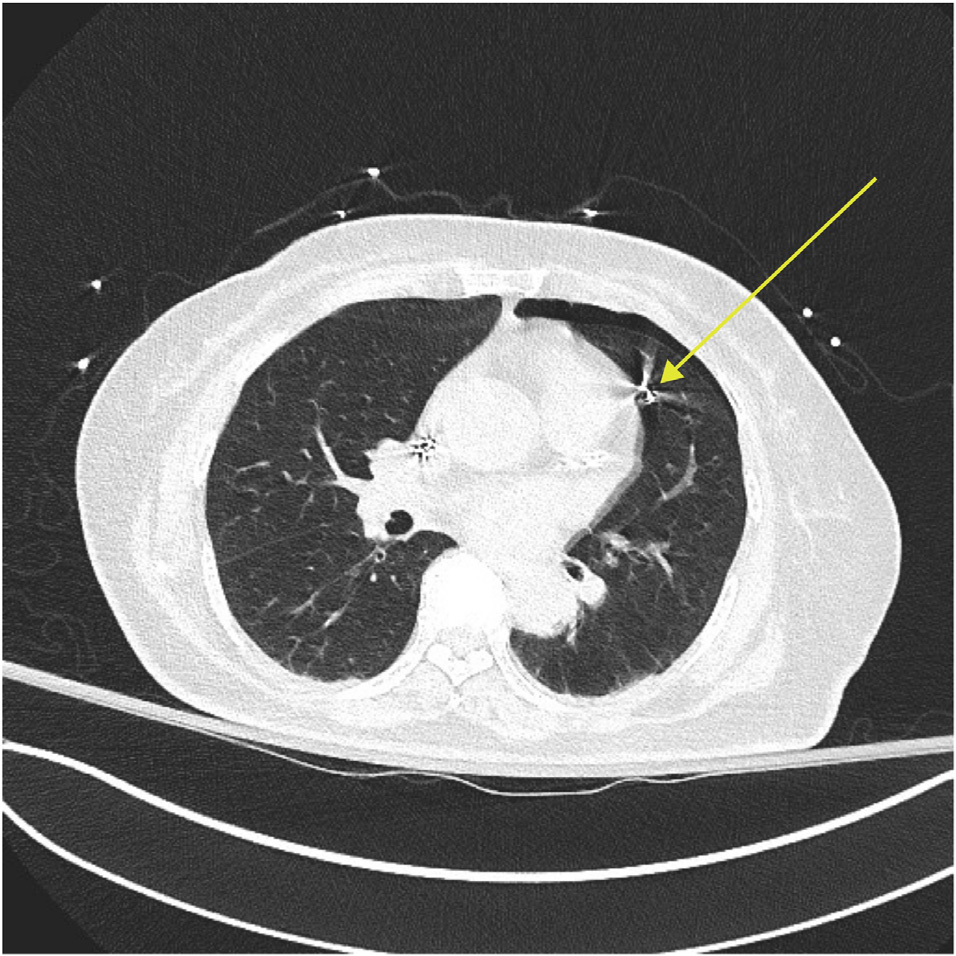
Lung window of the same gated CT angiogram of the chest, showing the left-sided apical pneumothorax. The right ventricular lead can be seen traversing through the pericardium and passing into the left upper lobe of the lung (yellow arrow).
